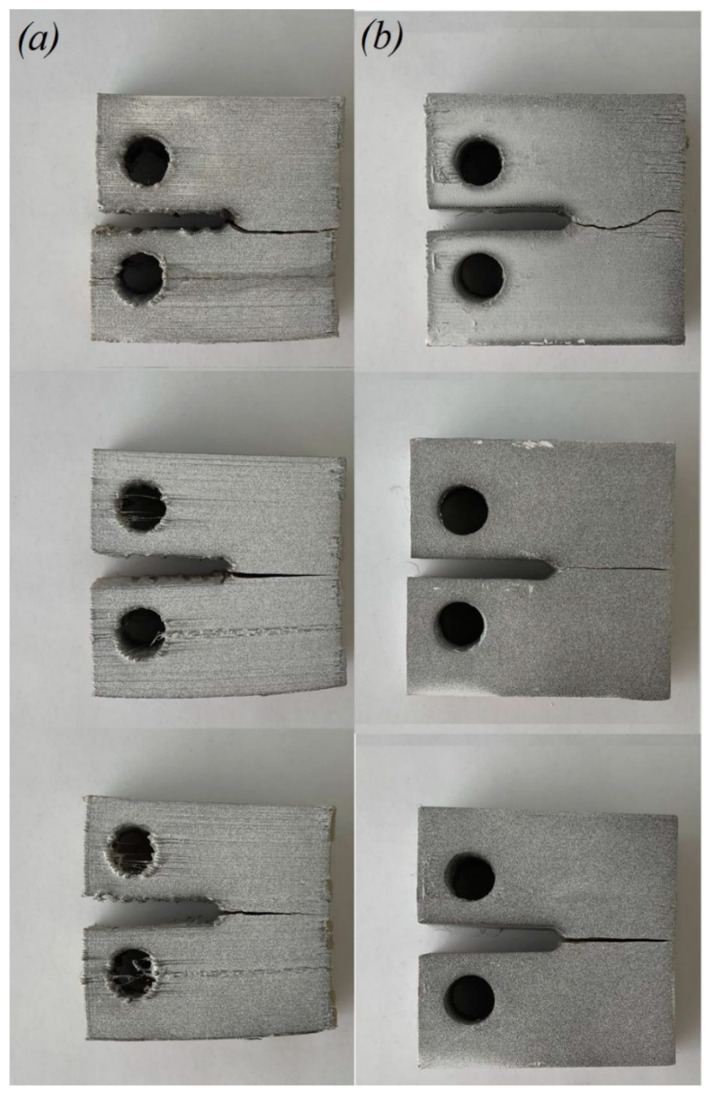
Fractured CT samples printed with vertical (a) and horizontal (b) orientations.
